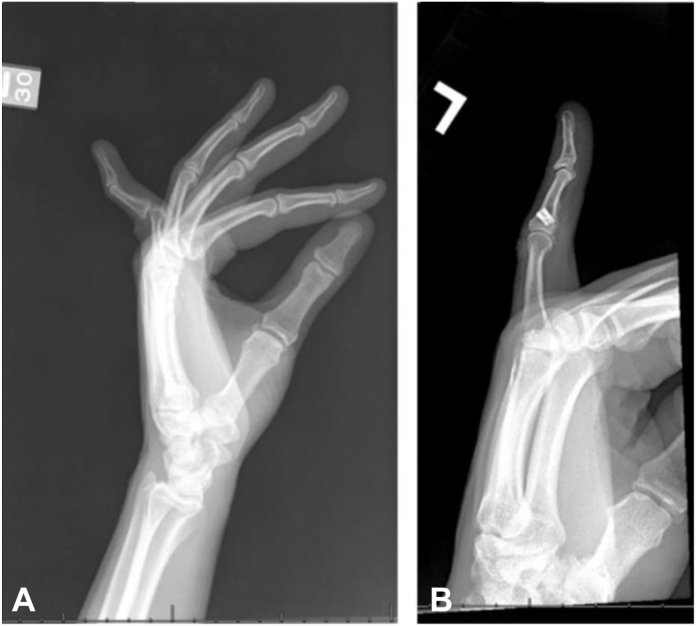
A Preoperative radiograph of a 30-year-old patient with a chronic VP injury. B An 11-year postoperative radiograph of a repaired VP 25 years after injury.
